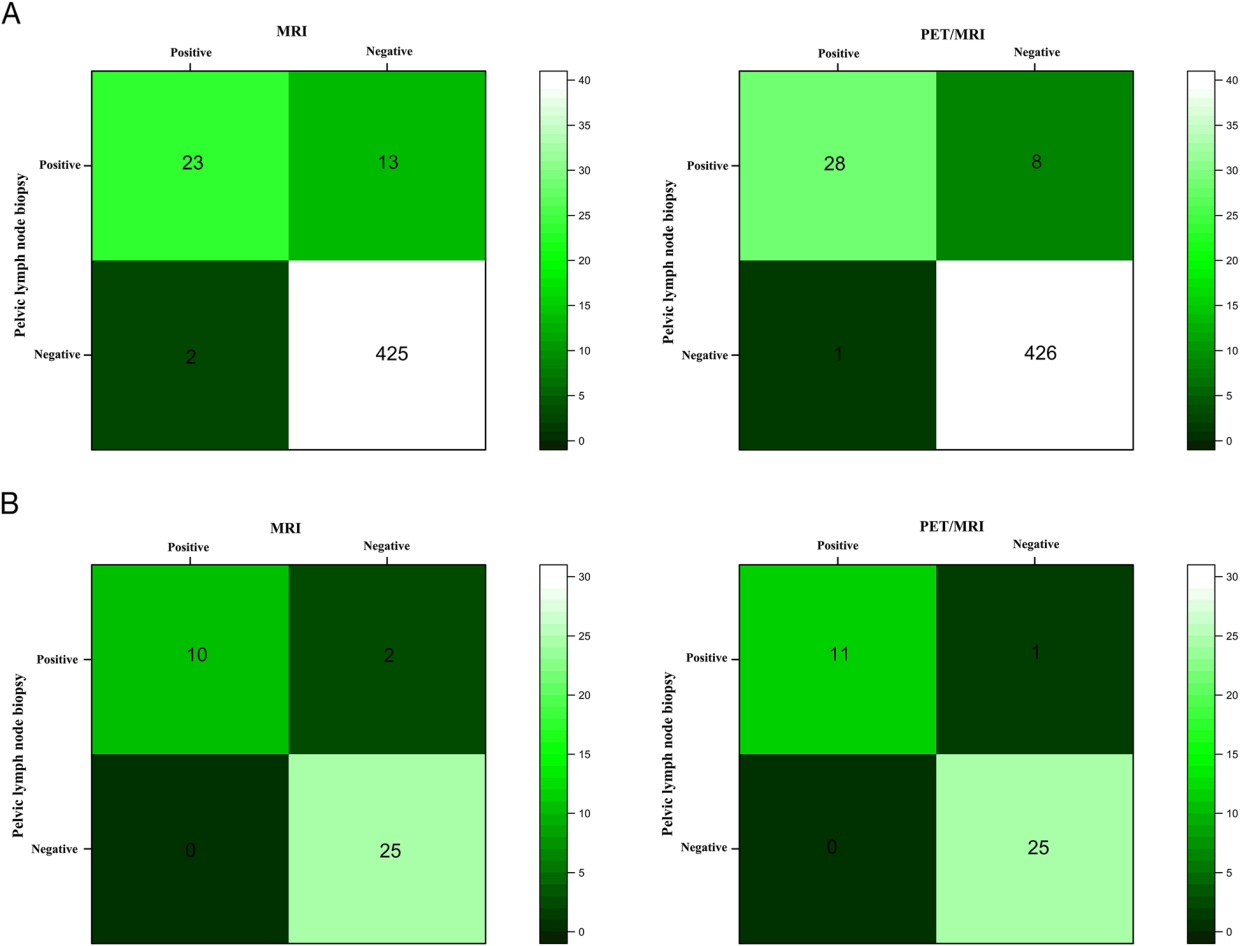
Confusion matrix results of MRI and PET/MRI for lymph node-based analysis (A) and for patient-based analysis (B)
